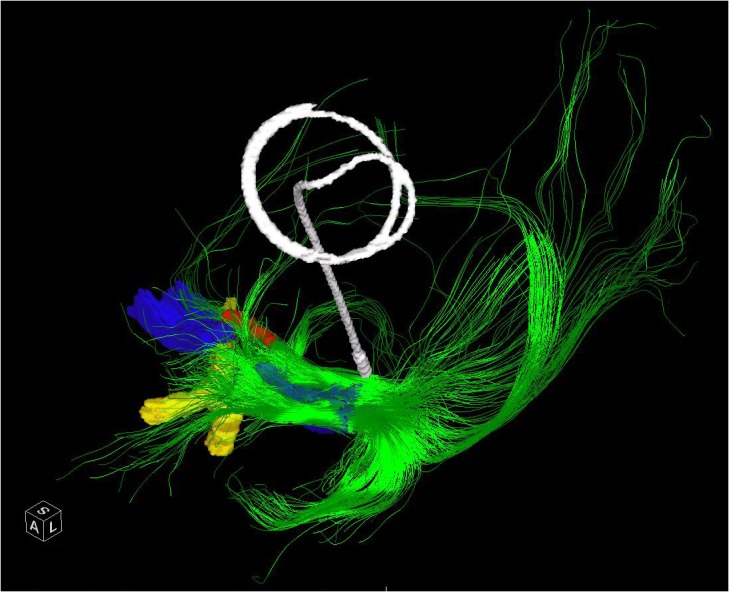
The entire left NBM has been seeded in a patient who subsequently underwent placement of a Left GPI DBS. The post-operative CT has been merged and the lead extracted to demonstrate the relative position of a standard GPI lead in relation to the bulk of the NBM outflow tracts. The bilateral NBM nuclei are reconstructed as described in the text and shown here in blue. The optic chiasm (yellow) and midline aspect of the anterior commissure (red) are shown for perspective. Frontal, temporal (via uncinate fasciculus) and parieto-occipital fibers are visualized.
